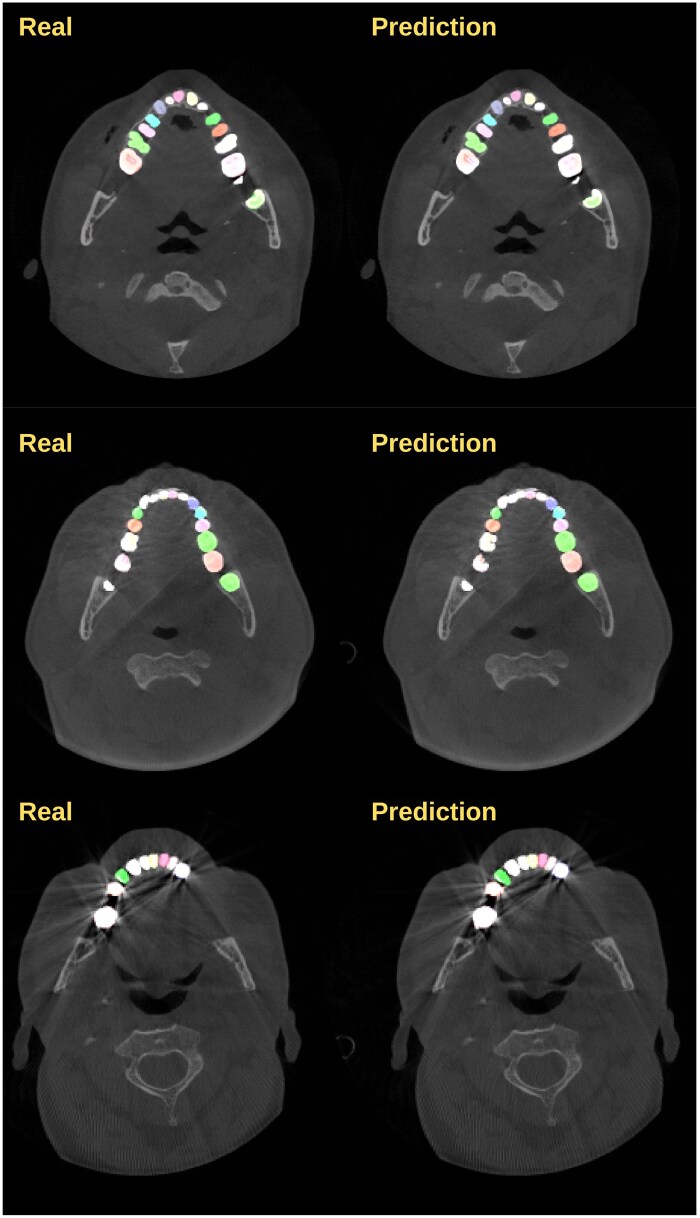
The automatic segmentation of the teeth utilizing the artificial intelligence model in axial CBCT slices.
